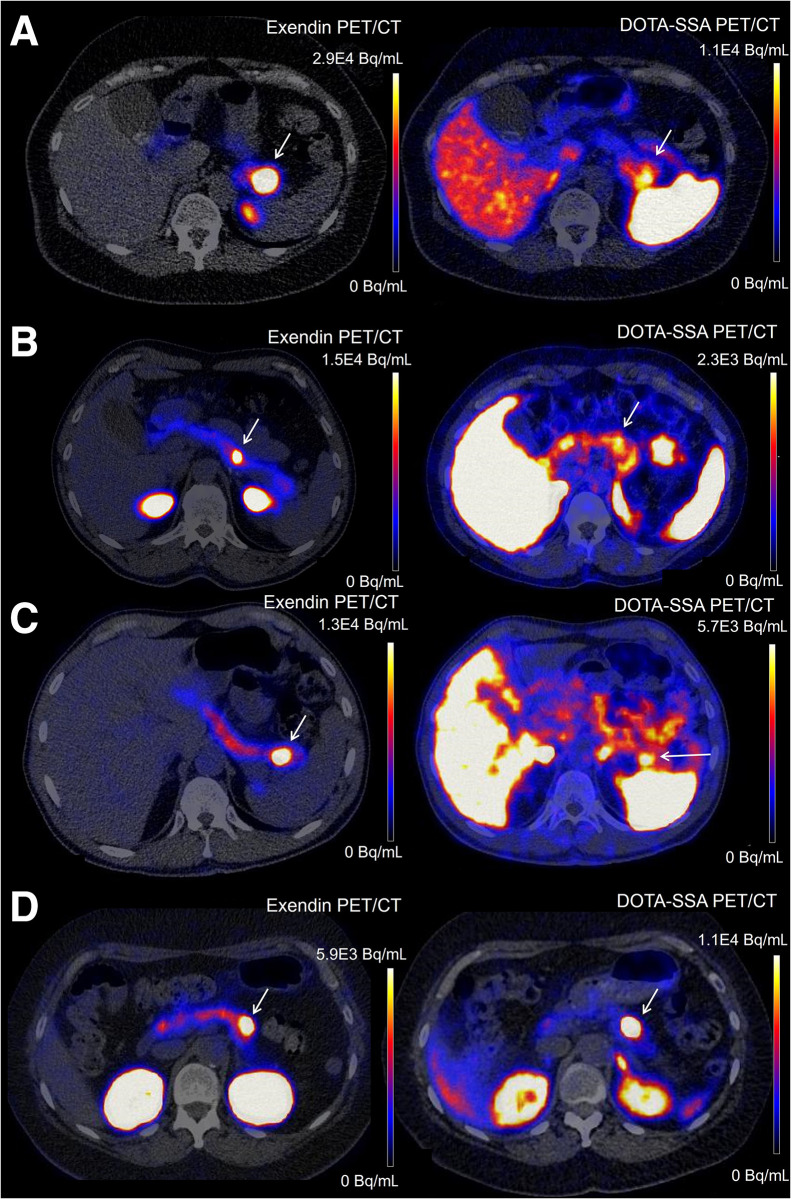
(A) Exendin PET/CT and DOTA-SSA PET/CT images of patient 56, with highest insulinoma-to-background ratio in exendin PET (31.5 vs. 2.3 in DOTA-SSA PET). Images of patients 4 (B) and 17 (C) show intermediate insulinoma-to-background ratios in exendin PET (8.9 and 10.4, respectively, vs. 2.2 and 2.3 in DOTA-SSA PET, respectively). (D) Images of patient 26 have lowest insulinoma-to-background ratio in exendin PET (7.3 vs. 12.2 in DOTA-SSA PET), showing still excellent visibility of insulinoma. Location of insulinomas is indicated with arrows.
